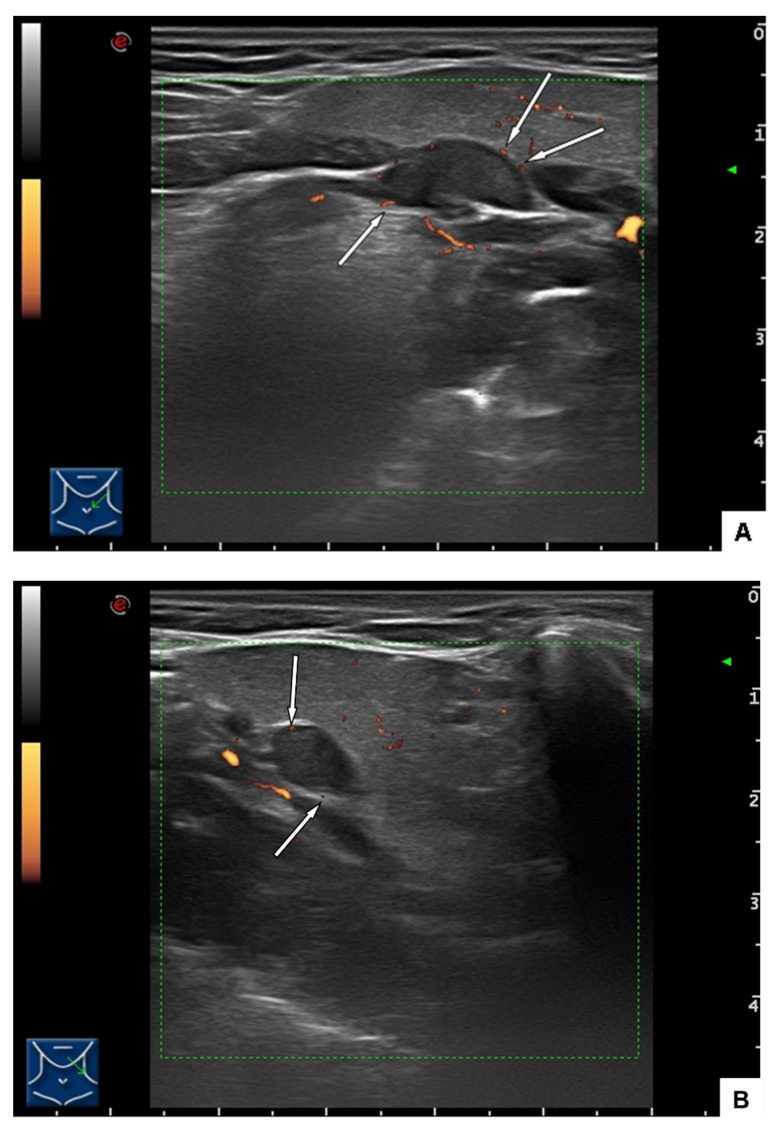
(A) Ultrasound findings: The rather homogeneous tumor is clearly demarcated from the surrounding tissues. Arrows indicate the position of vessels at the tumor surface. (B) Transverse view.
